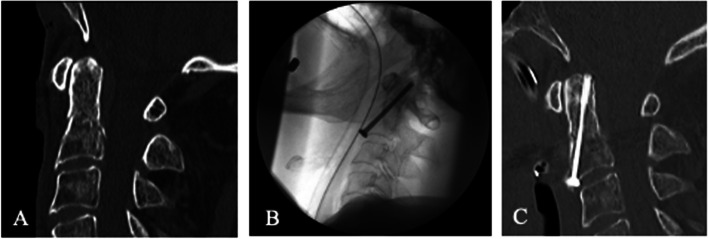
An 92–year–old male patient with odontoid fracture type A according to Eysel and Roosen. Based on the minor dislocation, ventral surgery was performed: Preoperative computer tomography (CT) image (A), intraoperative X–ray image (B), and postoperative CT image (C)
